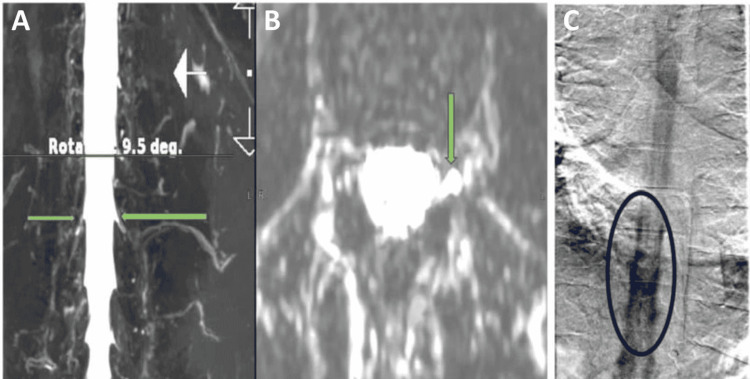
The thoracolumbar longitudinal and cross-sectional T2-SPACE MR myelogram images. (A and B) At the T11-T12 level, green arrows indicate fluid outside thecal space associated with a possible CSF leak. (C) DS myelogram confirms the localization of the CSF leak. Dye escaping the thecal sac can be observed at the level T12 to L1 (blue oval circle). The image is taken from Daripa B, Lucchese S: T2-SPACE protocol MRI: a safe, minimally invasive screening tool for spinal CSF leak causing spontaneous intracranial hypotension (P0253). J Headache Pain. 2021, 22:1-153. MR: magnetic resonance; CSF: cerebrospinal fluid; DS: digital subtraction
